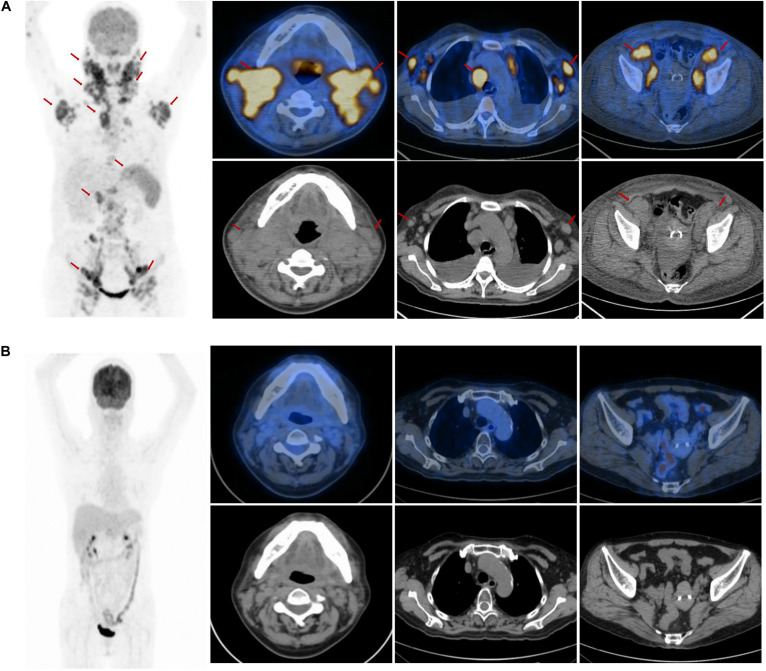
The positron emission tomography-computed tomography (PET-CT) scan at the first admission and the end of the salvage treatment. (A) The PET-CT scan at the first admission revealed elevated FDG uptake in multiple enlarged lymph nodes in the bilateral parotid gland, bilateral cervical, bilateral axillary, mediastinal, retroperitoneal, bilateral pelvic, bilateral inguinal regions, and in the nasopharynx, tonsil and spleen (arrow). (B) The PET-CT scan showed no elevated FDG uptake lesions at the end of the salvage treatment.
